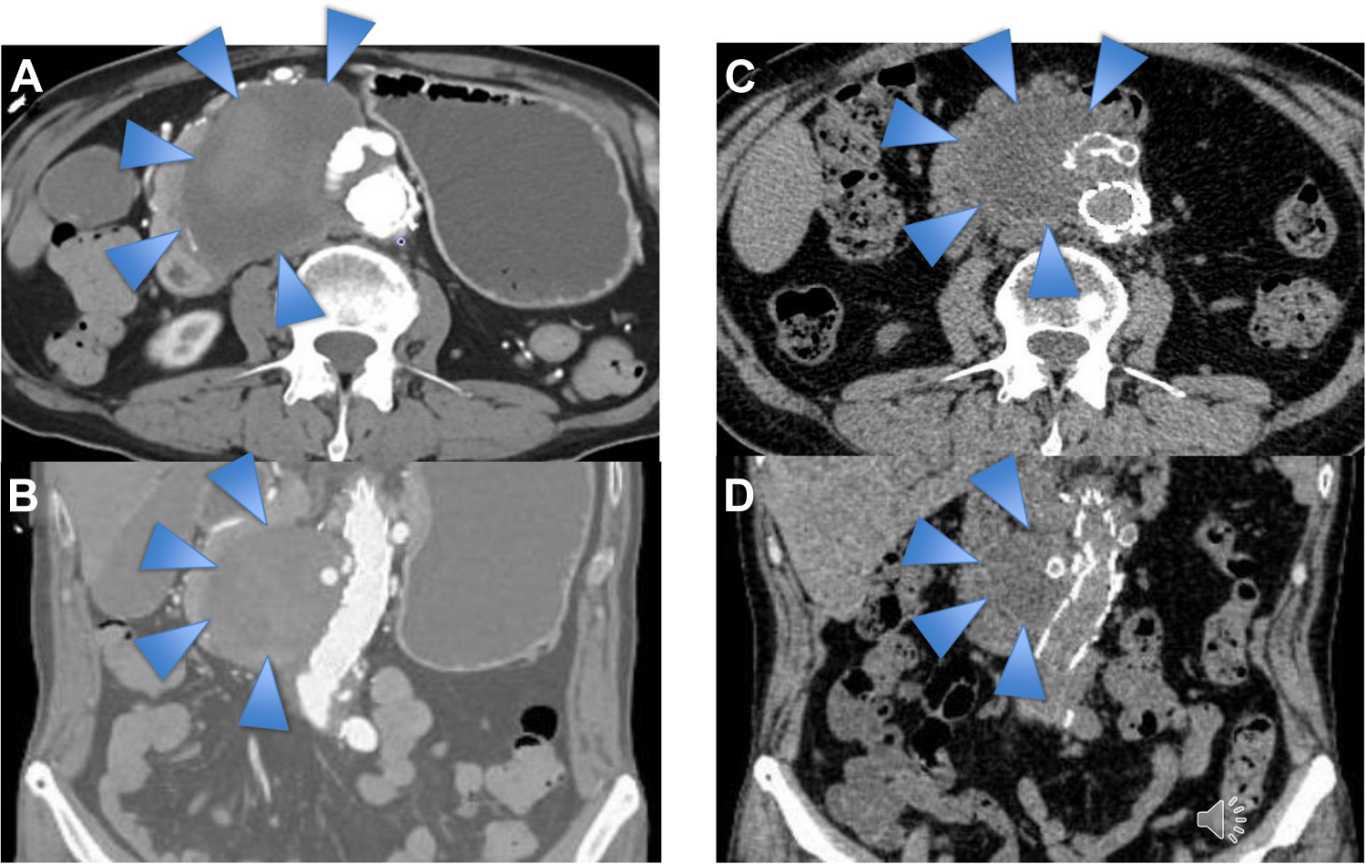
Computed tomography images at admission and 3 months after endovascular repair. A, Axial view at admission. B, Coronal view at admission. C, Axial view at 3 months after endovascular repair. D, Coronal view at 3 months after endovascular repair. The obtained images showed that the seroma (*blue arrows*) had decreased in size from 95 × 60 to 45 × 30 mm within 3 months after endovascular repair.
